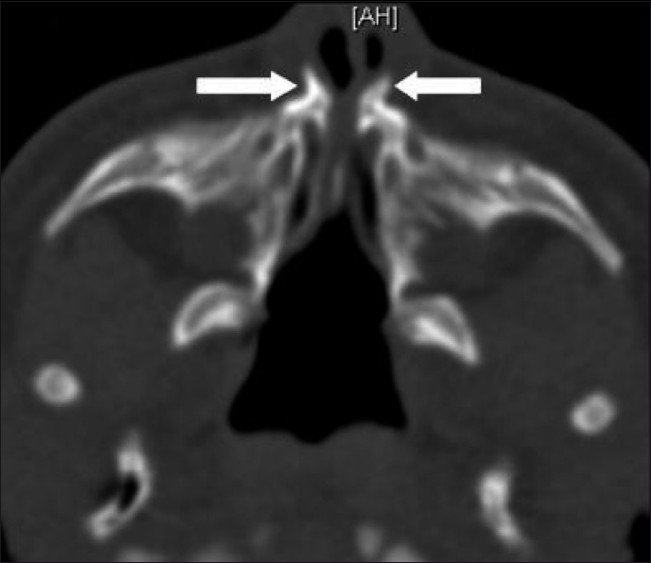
Axial CT scan shows medial approximation of the nasal processes of the maxilla (arrows) causing marked pyriform aperture narrowing
